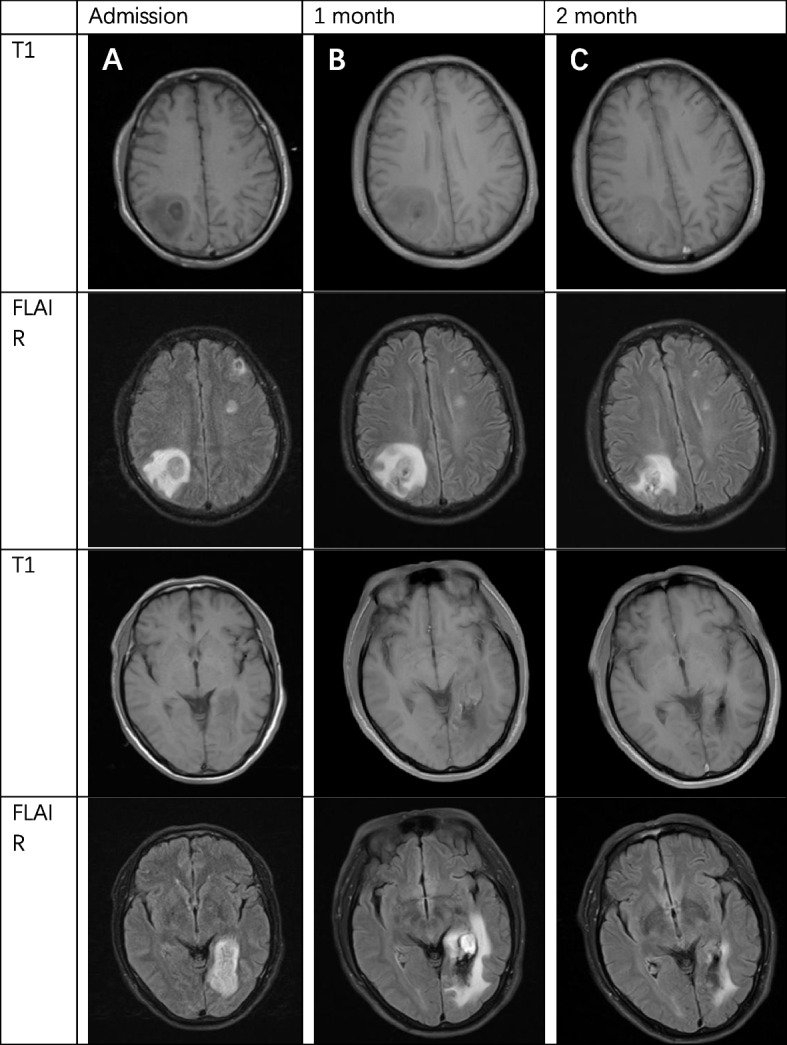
MRI of brain abscess changes during treatment. A-C (from onset to 2 months) showed progressive reduction of cerebral lesions with prolonged treatment
